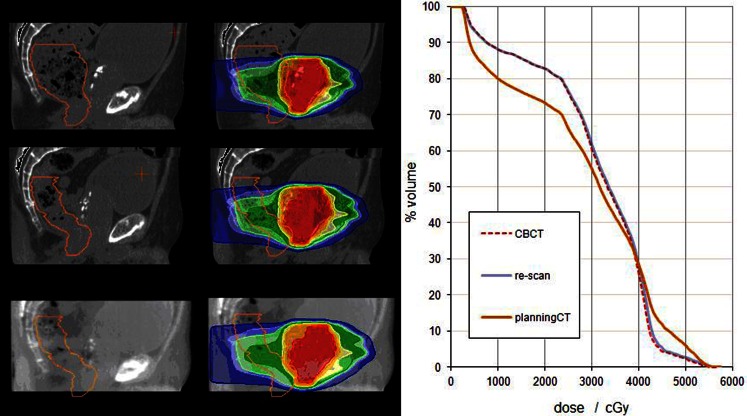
Left*: Sagittal images of a prostate RT treatment plan with dose calculated on (*top*) the PCT_orig_; (*middle*) PCT_CBCT_; and (*bottom*) the CBCT. The rectum is shown as an *orange contour* and the dose is shown in color wash relative to the prescribed dose. *Right:* dose–volume histogram of the rectum for the dose calculated on the PCT_orig_ scan (*orange line*); the PCT_CBCT_ (*blue line*); and the CBCT (*red dashed line*). The RS_auto_ method was used for CTN adjustment of the CBCT image. The rectum OAR was similar in size and shape on the CBCT and the PCT_CBCT_ but was very different to that seen on the PCT_orig_ scan. *PCT* planning computed tomography, *CBCT* cone beam CT, *CTN* CT number, *OAR* organ at risk
